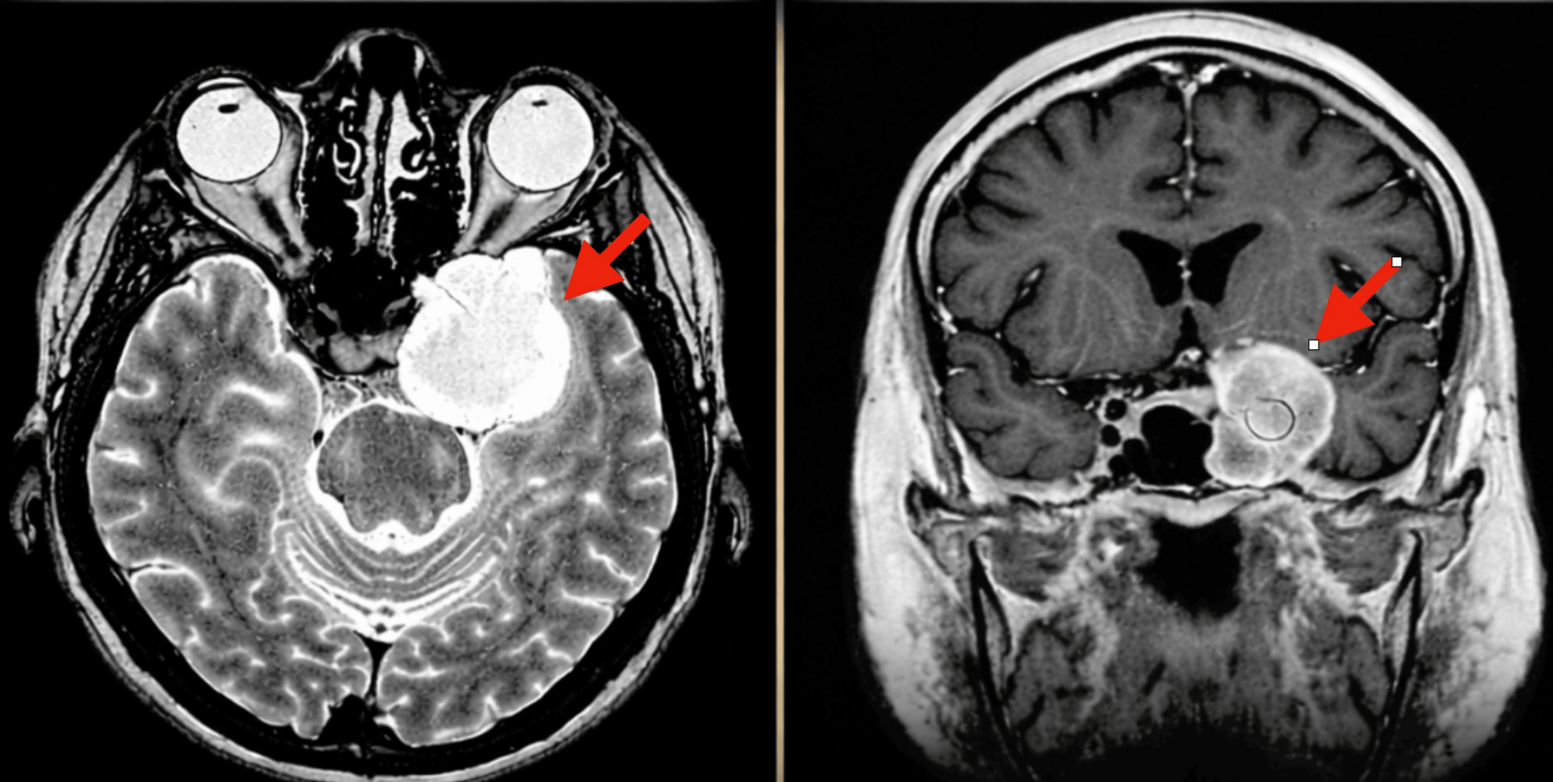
Axial and Coronal MRI Demonstrating a Left Cavernous Sinus Cavernous Angioma Axial T2-weighted (left) and coronal T1-weighted post-contrast (right) MRI images revealing a well-defined, expansile lesion centered within the left cavernous sinus. The mass demonstrates smooth contour expansion with displacement of adjacent parasellar structures, characteristic of a cavernous sinus cavernous angioma (red arrows).
